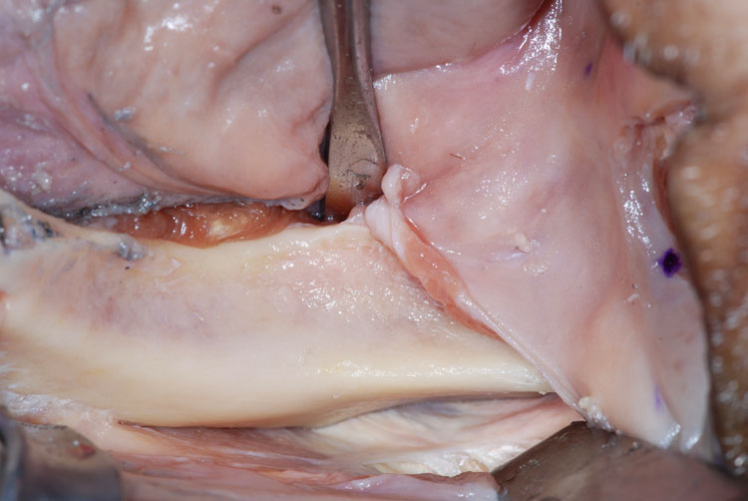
The harvesting area was selected after a careful assessment on the CBCT. The area was widely exposed after the reflection of a mucoperiosteal flap, from the first molar to all extension external oblique line of the mandible.
